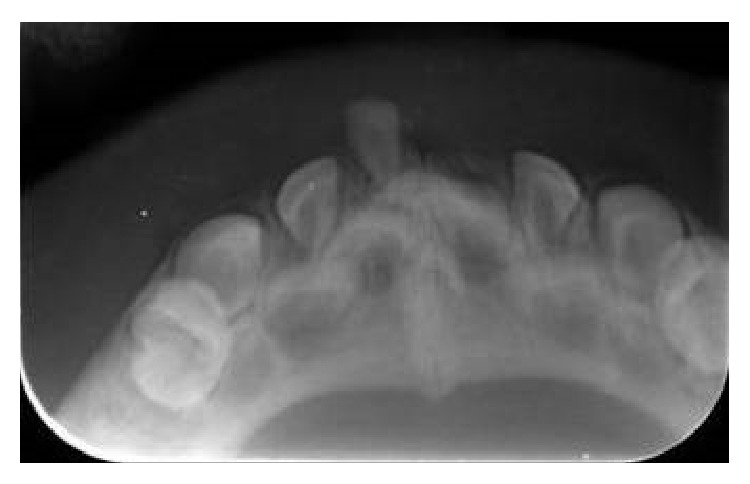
Radiographic aspect of the patient at one year of follow-up showing that the natal teeth were regular primary teeth.
